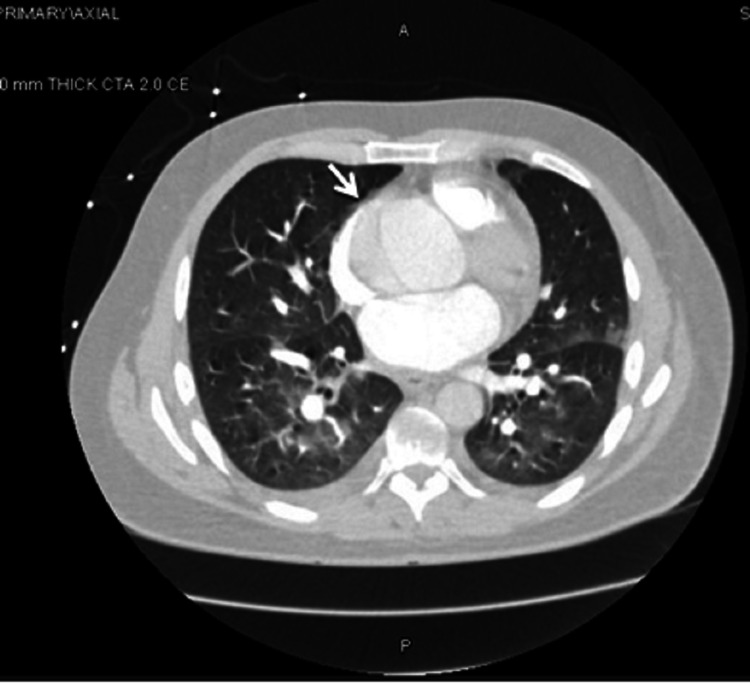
Axial CT angiography of the thorax showing aortic dissection in ascending and descending aorta, aneurysmal dilatation (arrow) of the ascending thoracic aorta, and diffuse patchy bilateral nodular infiltrates
